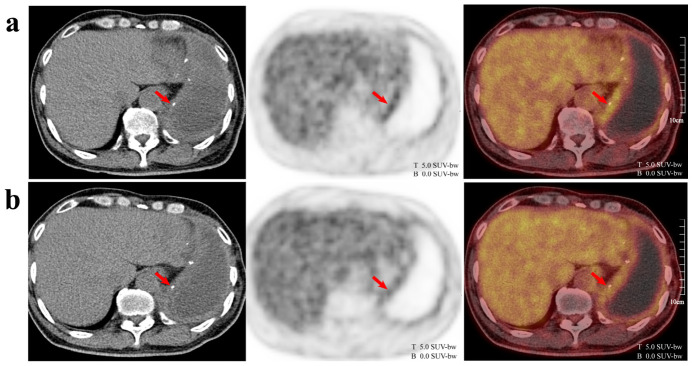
Time dependent reduction in postsurgical FDG uptake. A 64-year-old man underwent extrapleural pneumonectomy for malignant pleural mesothelioma. ^18^F-fluorodeoxyglucose (FDG) positron emission tomography/computed tomography (PET/CT) scans at 12 months (a) and 22 months (b) postoperatively showed a progressive decrease in FDG uptake within the surgical bed over time (arrows). Spatial scale bars (10 cm) and FDG uptake intensity scales (0.0–5.0 SUV-bw) are displayed for reference. T and B indicate the top (maximum, 5.0 SUV-bw) and bottom (minimum, 0.0 SUV-bw) of the FDG uptake intensity scale, respectively.
